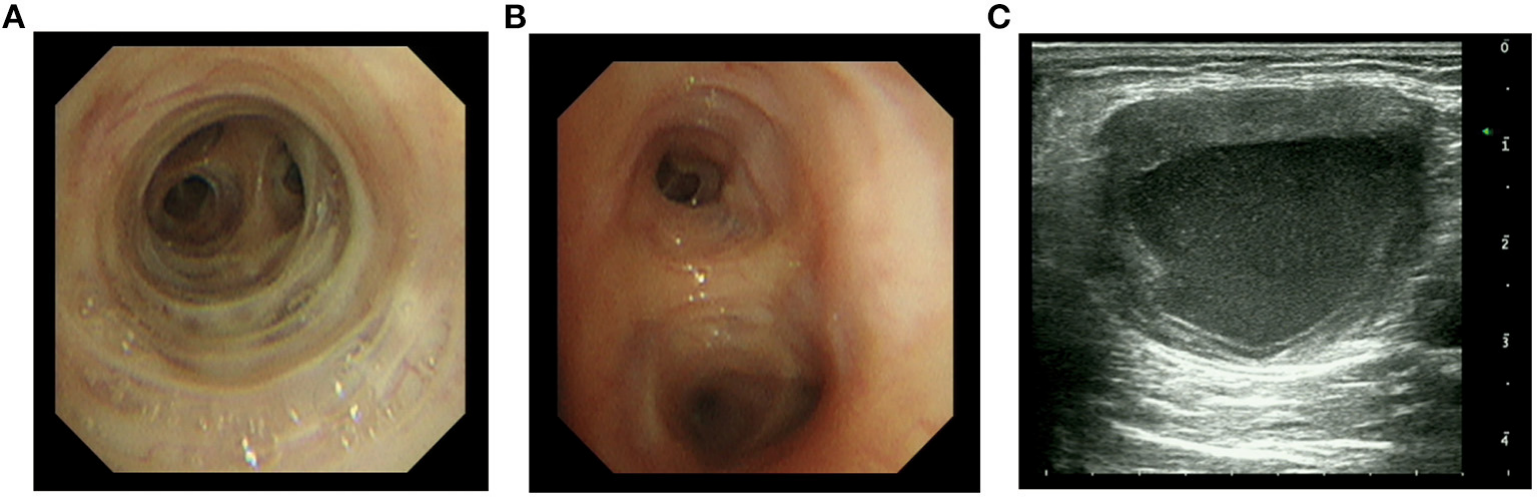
Bronchoscopy and ultrasound imaging of an abscess. (A,B) Right middle and left lower bronchoscopy, respectively. (C) Ultrasound imaging of an abscess in the left upper arm.
